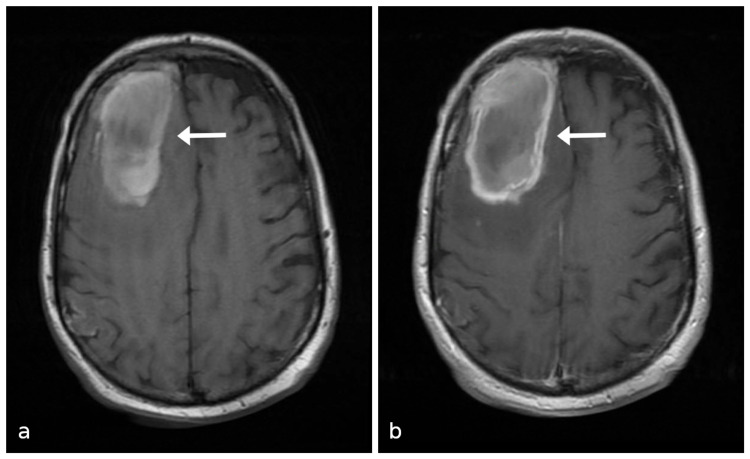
(a) MRI T1 pre-contrast and (b) post-contrast sequences demonstrating right frontal ring-enhancing lesion. MRI, magnetic resonance imaging
